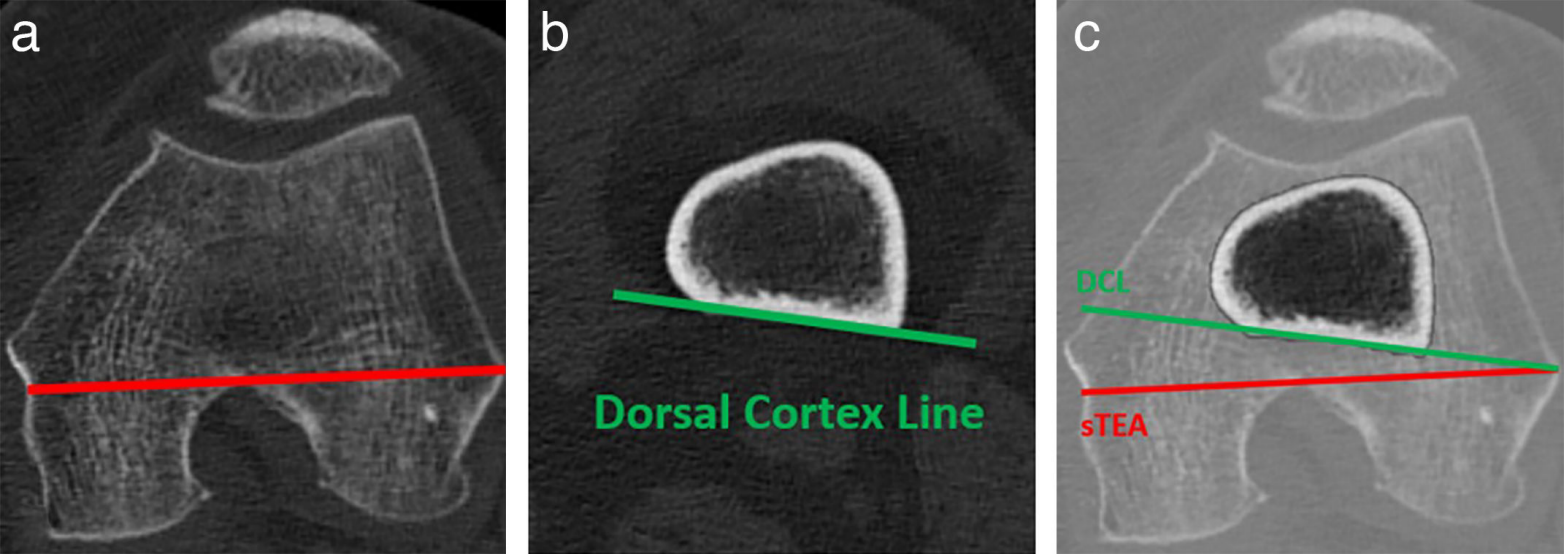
a) Surgical transepicondylar axis in a coronal CT plane; b) dorsal cortex line measured 75 mm above the joint line in coronal plane; and c) an example of overlap between surgical transepicondylar axis and dorsal cortex line.
